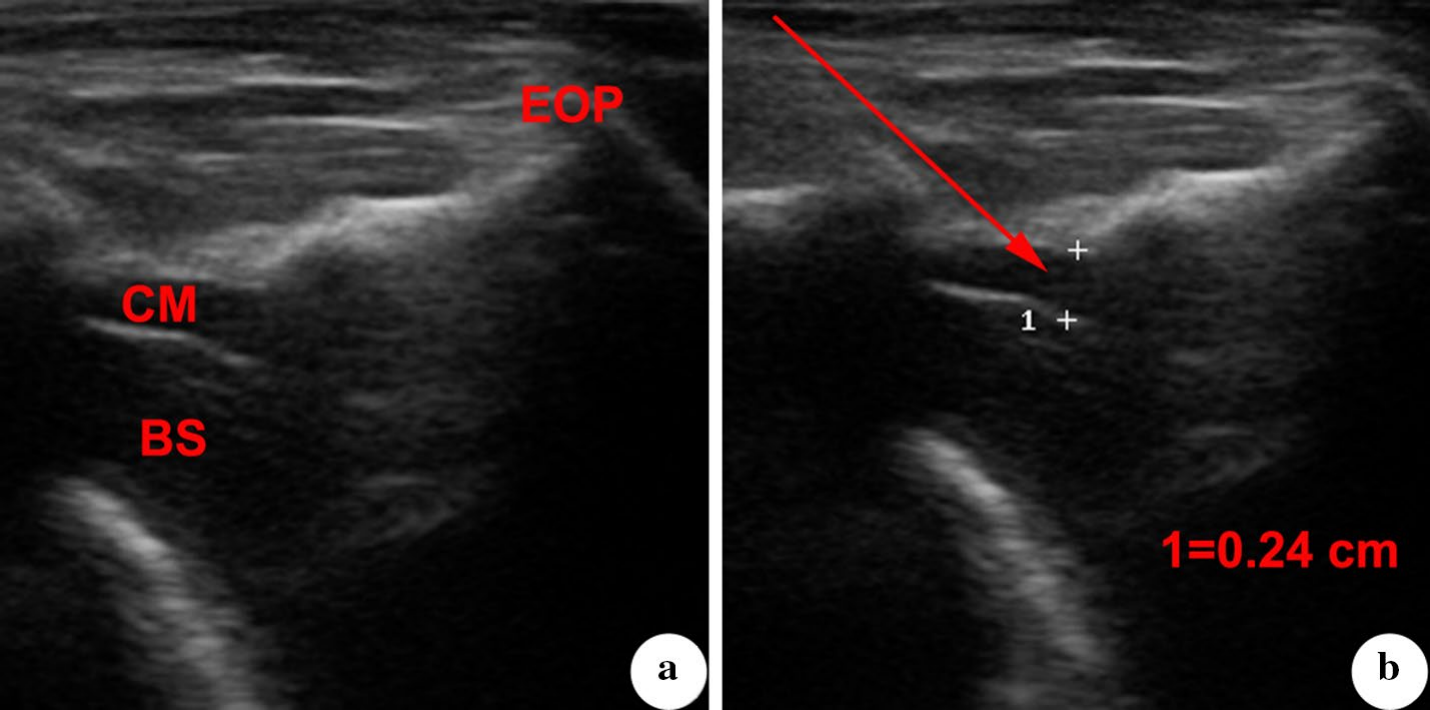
Measurement and observation of cisterna magna that is guided by ultrasound. a Cisterna magna and adjacent structures were displayed by ultrasound. b Depth of cisterna magna was measured at its optimal part. The puncture direction was designed based on the *line* from atlano-occipital fascia to the deepest part of cisterna magna. *CM* cisterna magna, *BS* brain stem, *EOP* external occipital protuberance
